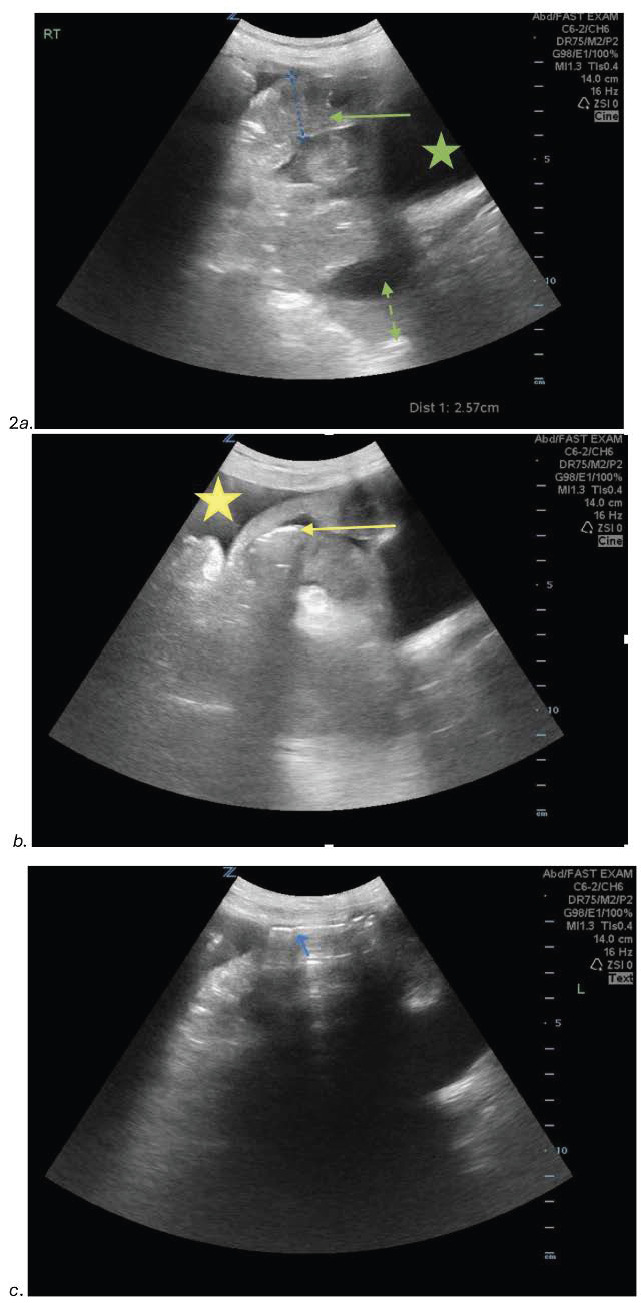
(a): A point of care ultrasound (POCUS) image of the abdomen with a curvilinear probe showing a dilated loop of small bowel (arrow) with free fluid (dashed arrow), and a visualization of the bladder (star). (b): A point of care ultrasound (POCUS) image of the abdomen showing dilated loops of small bowel with evidence of hyperechoic foci within the bowel wall (arrow) and the presence of free intra-abdominal fluid (star). (c): A point of care ultrasound (POCUS) image of the right upper quadrant in sagittal orientation showing a-lines within the abdomen (arrow) with reverberation artifact.
